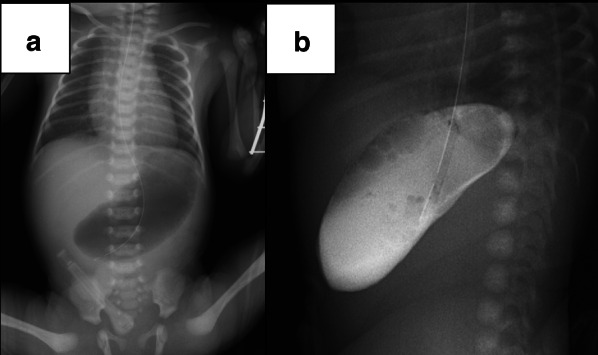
Radiological findings of Case 1: a plain radiograph showing dilated stomach and absence of gas in the duodenum, distally; b upper gastrointestinal contrast study showing dilated stomach and complete gastric outlet obstruction
